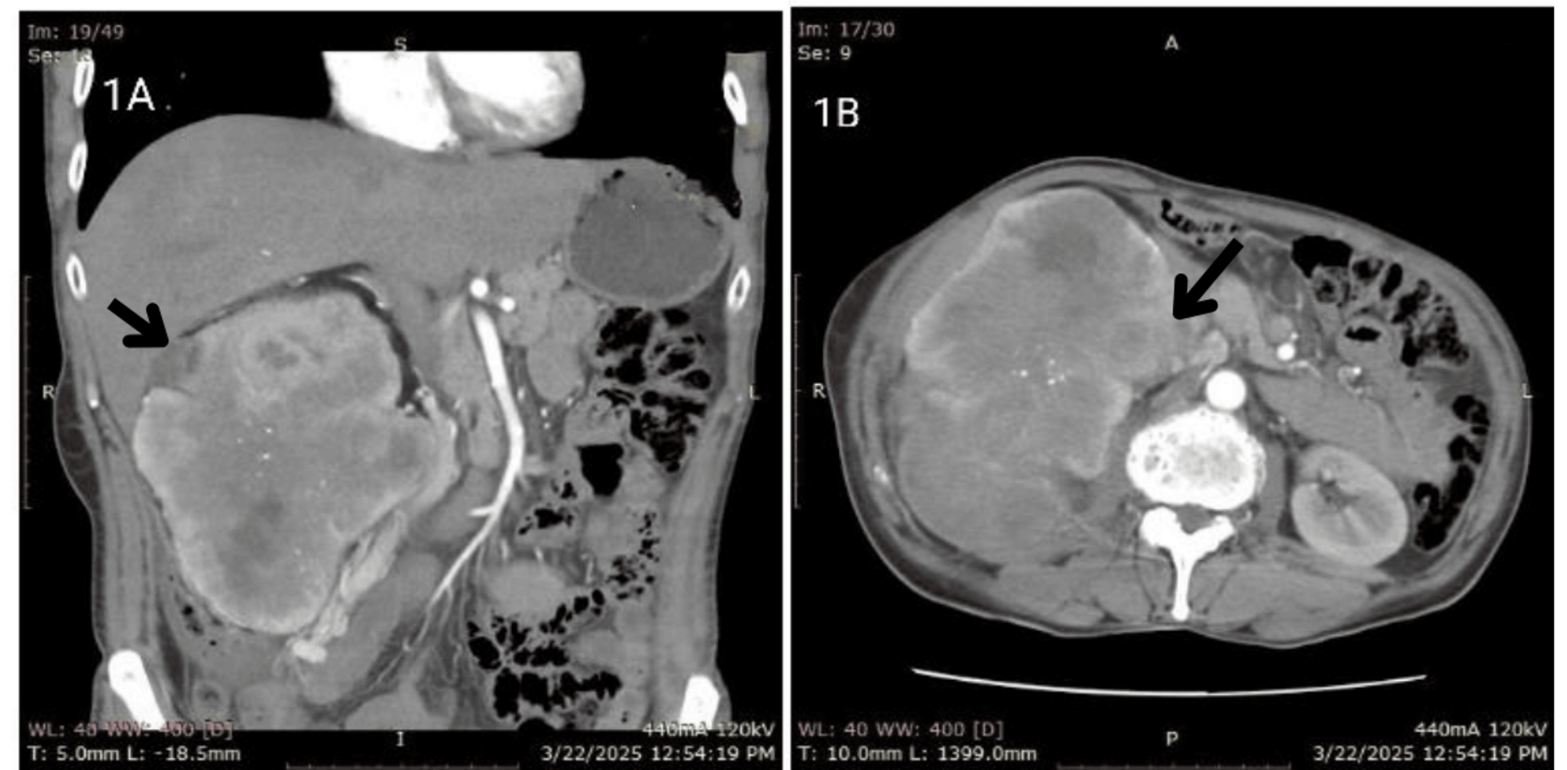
CECT abdomen and pelvis showing a large, well-defined heterogeneous mass originating from the lower pole of the right kidney exhibiting mixed density with fatty components, cystic degeneration, and coarse calcifications (1A) Axial view; (1B) coronal view. CECT, contrast-enhanced computed tomography.
